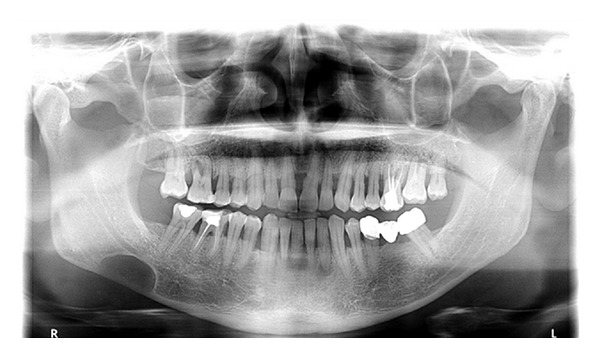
Panoramic radiography showing the radiolucent area.
